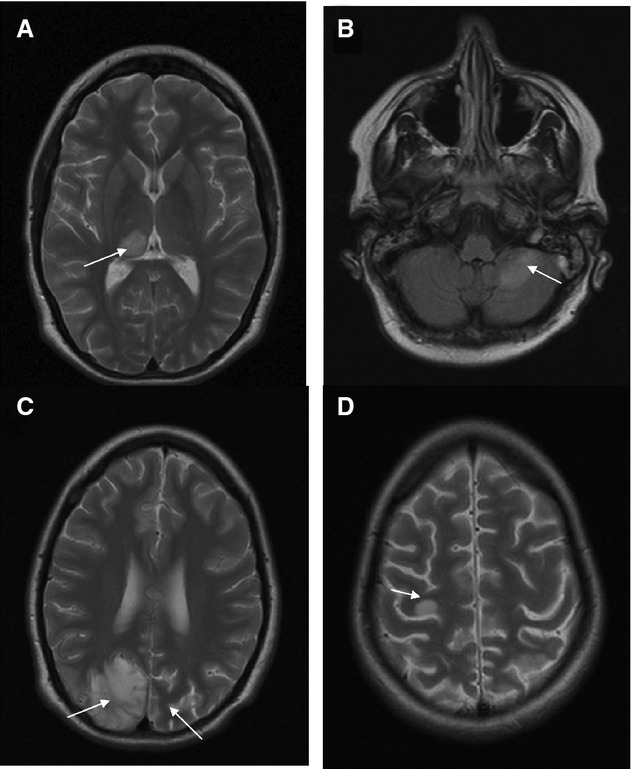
Progressive changes in brain MRI with continued fitting associated with *POLG* mutations. The brain MRI at presentation with the first focal epileptic seizure of patient 7 with a homozygous p.W748S/p.W748S was normal (not shown). On admission 1 year later a clearly circumscribed region of abnormal high signal intensity (SI) was seen in the right posterior thalamus on T_2_-weighted and FLAIR images (A, axial T_2_-weighted image shown at the level of the basal ganglia) and abnormal low SI on T_1_-weighted imaging (not shown). Lesions of similar SI were seen in two sites in the left cerebellar hemisphere (B, axial FLAIR image). Two months later, bilateral parietal-occipital lesions involving the white matter and cortex (right much more than left) were seen (C, axial T_2_-weighted image) with improvement in the previous abnormalities without atrophy, although the abnormal SI in the left thalamus remained obvious. A further scan 3 months later showed new lesions in the cortex (D, axial T_2_-weighted image, arrow).
